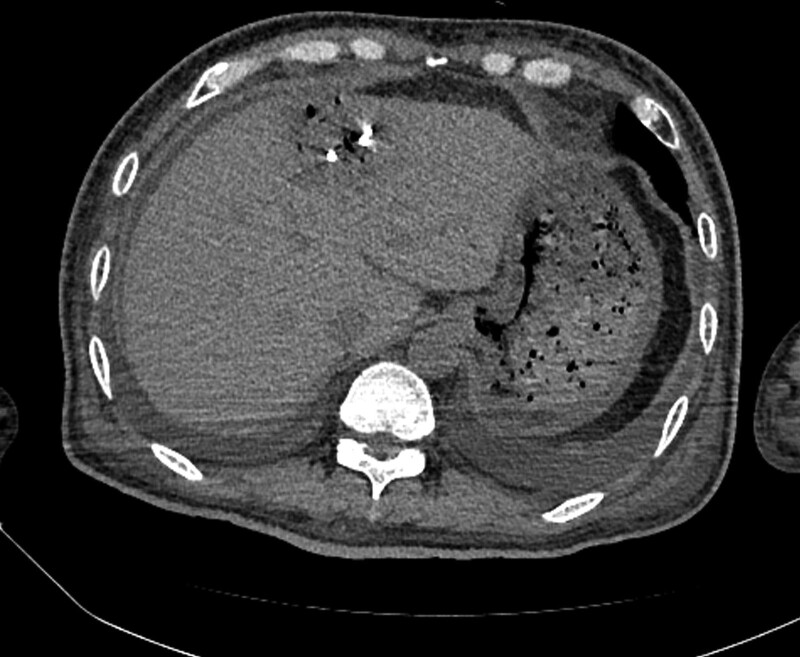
Plain computed tomography 6 days after the initial examination showed an apparent reduction of lesion range and air collection after percutaneous catheter drainage.
